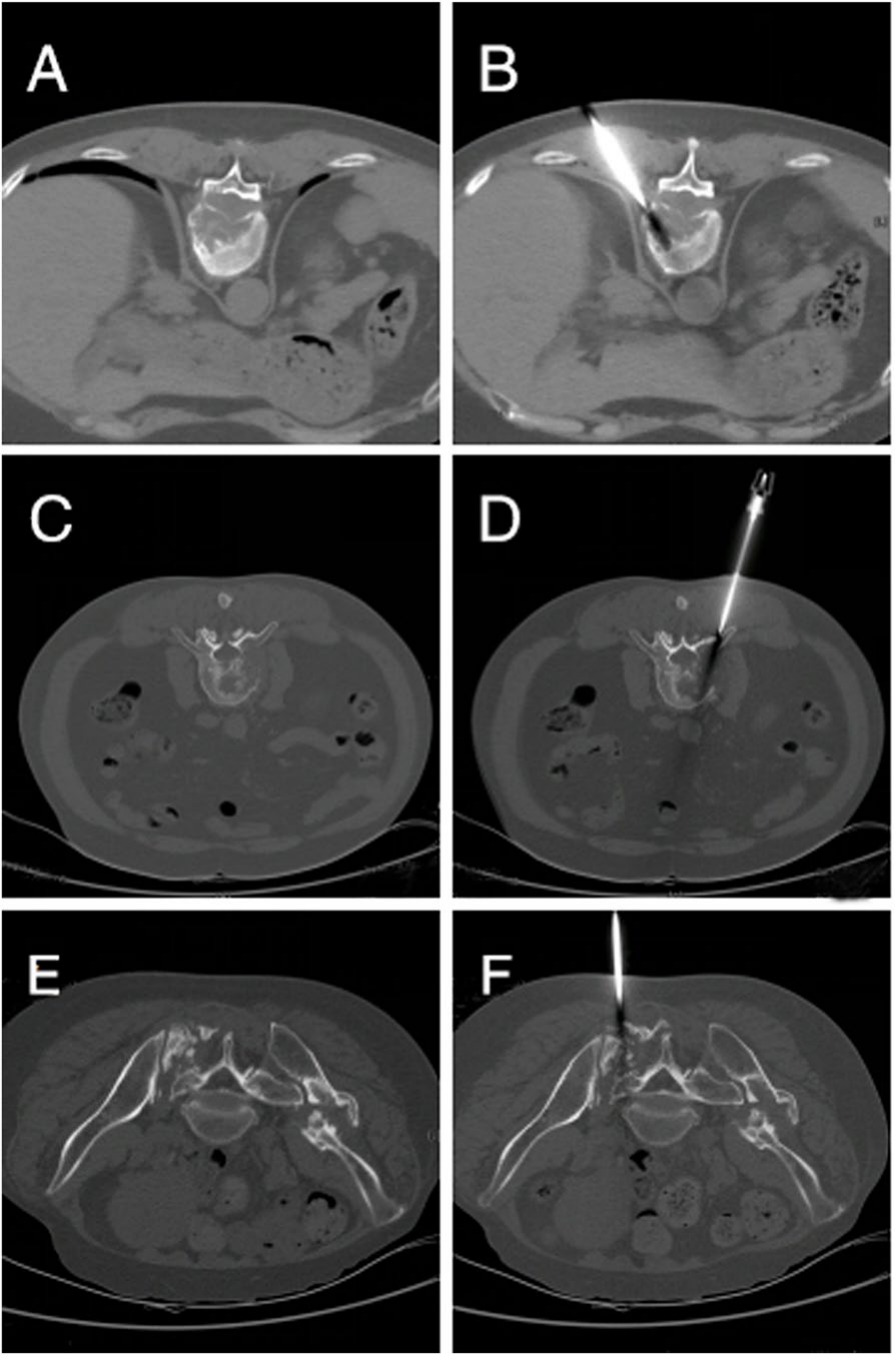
Vertebroscopic biopsy under CT guidance. A and B. Puncture biopsy of a solitary plasmacytoma in the eleventh thoracic vertebra; (C and D) Puncture biopsy of an undifferentiated pleomorphic sarcoma of the body of the third lumbar vertebra; (E and F) Puncture biopsy of a sacral metastasis of a right kidney clear-cell carcinoma
